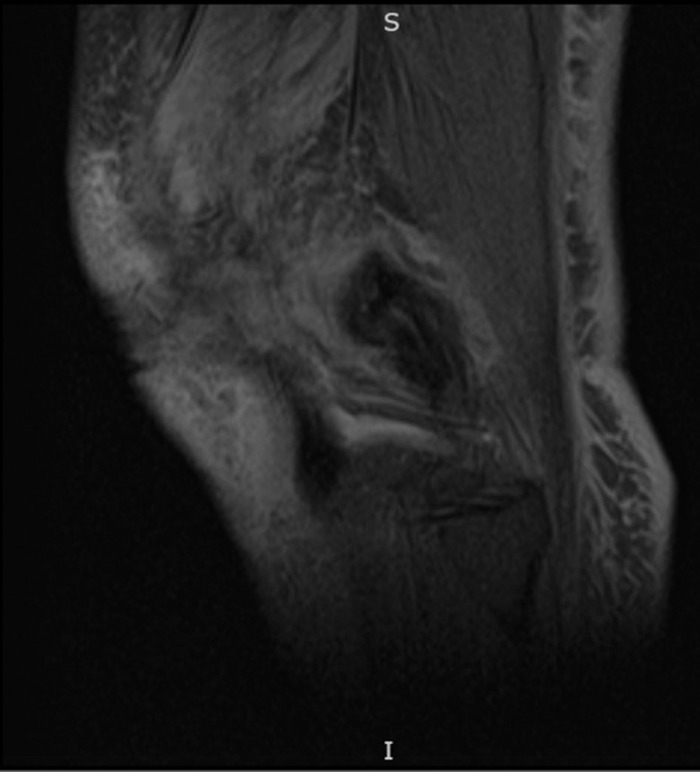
Cellulitis noted on magnetic resonance imaging corresponds with the wound shown in Figure 3.
